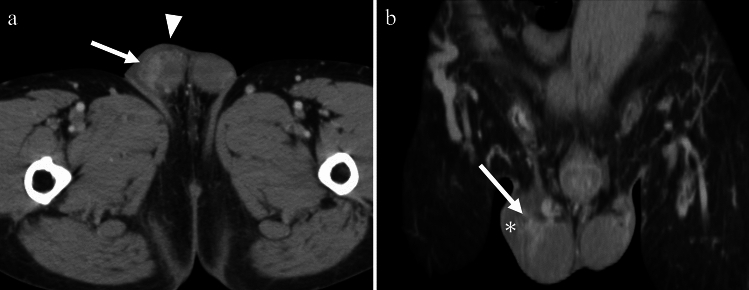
Man in his 30 s with Bechet’s disease presenting with right scrotal pain a, b On contrast-enhanced CT in axial and coronal sections, there is enlargement and strong enhancement of the right epididymis (arrows), accompanied by thickening of the scrotal wall (arrowhead in a) and reactive hydrocele (asterisk in b), indicating epididymitis
